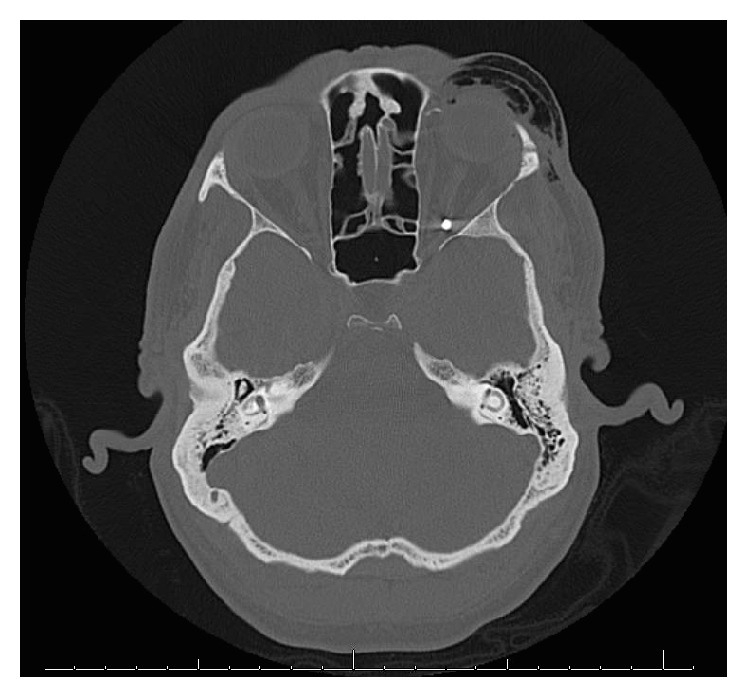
A representative axial head computed tomography image demonstrating an intraorbital metallic foreign body adjacent to the left optic nerve.
